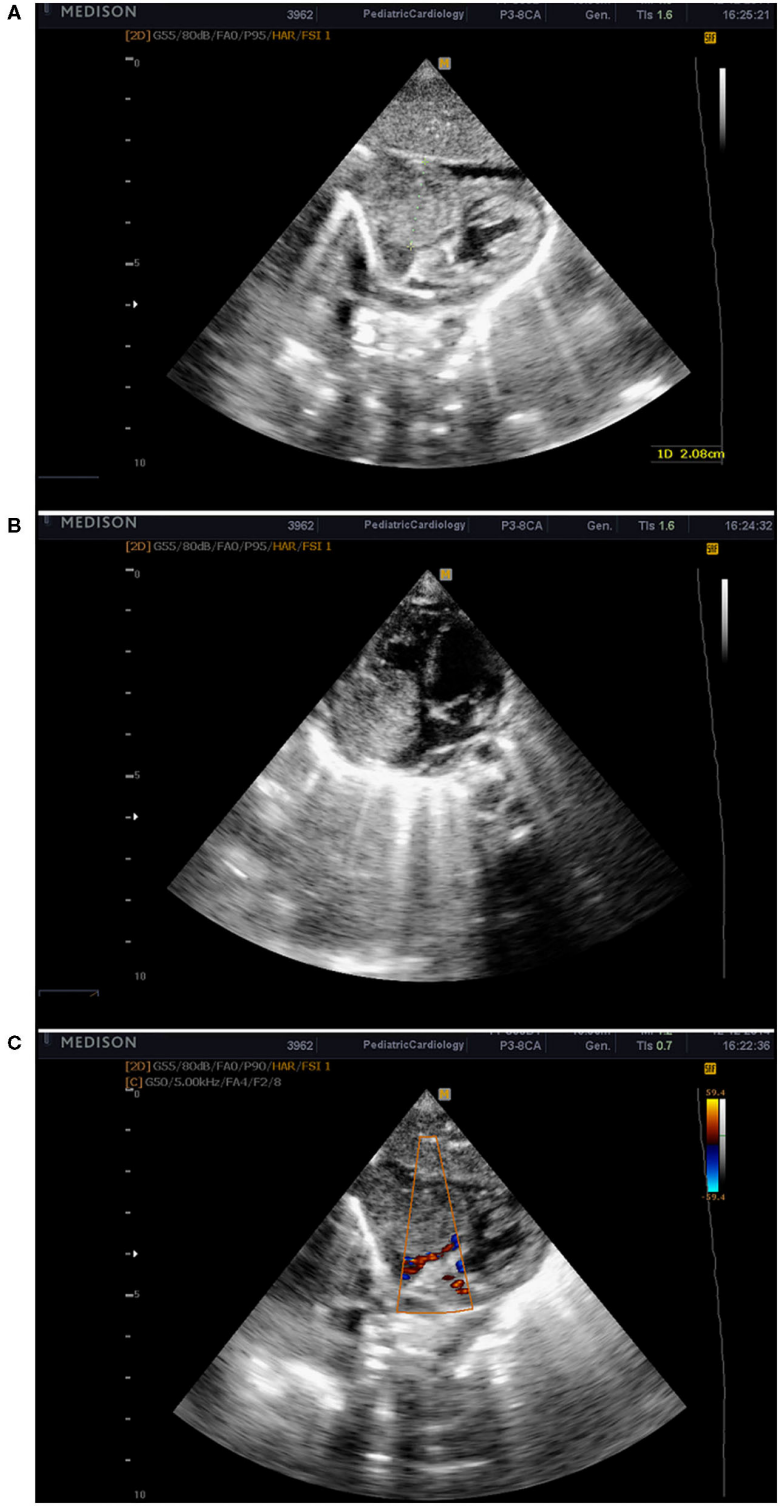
Echocardiographic images from the subcostal window showing the tumor mass in the right atrium (A,B). Obstruction in the output of the right atrium and reduced blood flow to the right ventricle (C).
